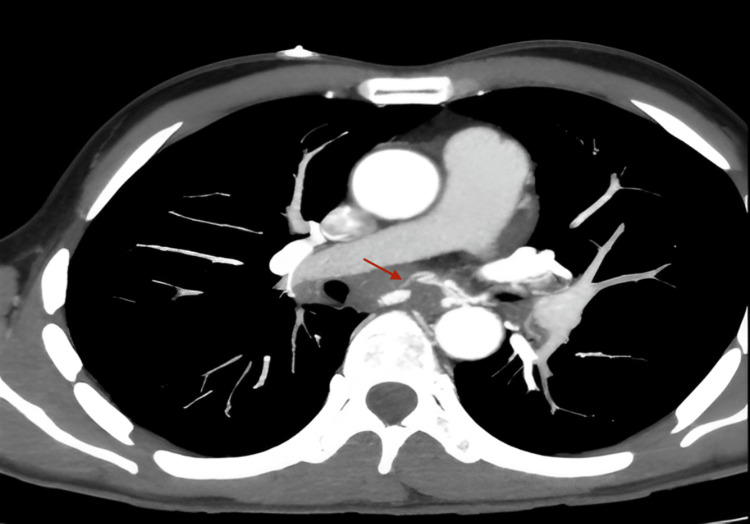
CT scan without injection in the arterial phase showing esophageal bleeding. This is an axial contrast-enhanced CT slice at the level of the pulmonary arteries. The red arrow points to a focal area of contrast leakage adjacent to a pulmonary artery. This hyperdense area outside the vessel indicates active hemorrhage.
